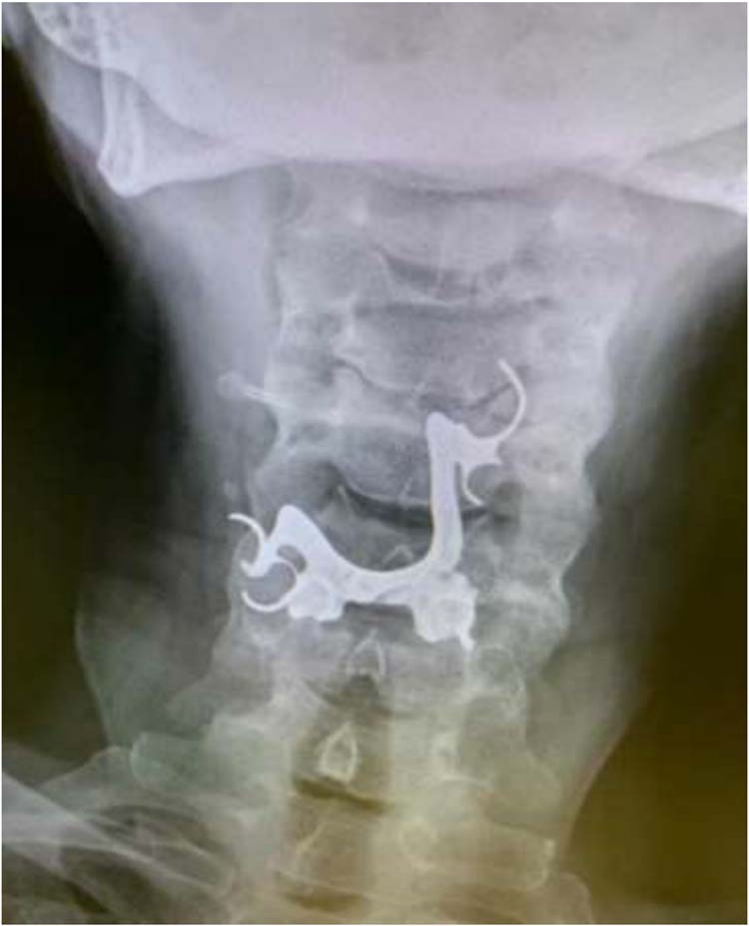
Anterior-posterior neck X-ray showing a radio-opaque object, in keeping with metal dentures.
